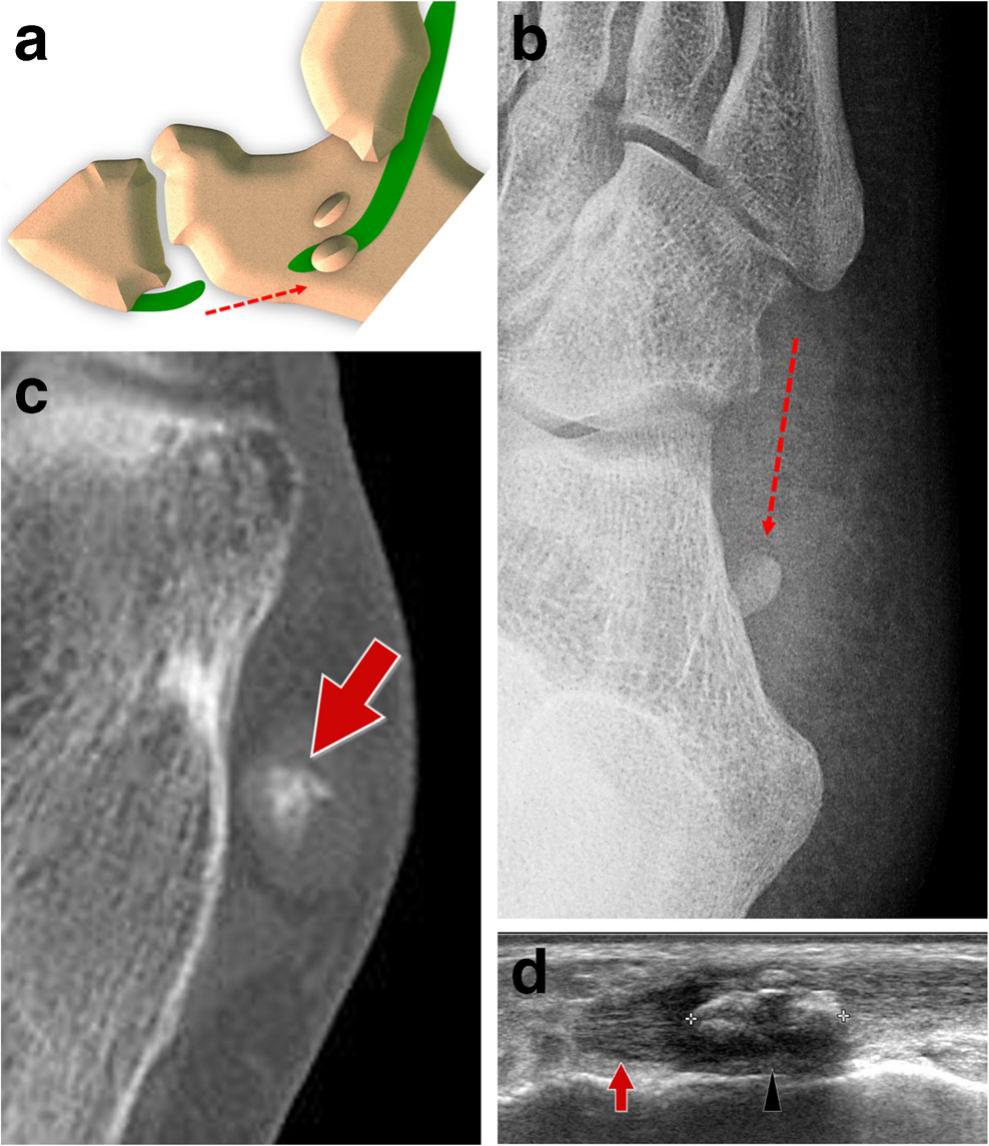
PLT tear type III standard retraction. *14A* Scheme *14B* Standard internal oblique radiograph. The OP shows a normal appearance but is posteriorly dislocated (generally less than 2 cm). *14C* Computed tomography. CT shows a regular appearance of the displaced sesamoid bone. *14D* Ultrasound. The degree of the dislocation and the size of the proximal tendinous stump (*arrows*) are easily measured. OP (*arrowhead*)
